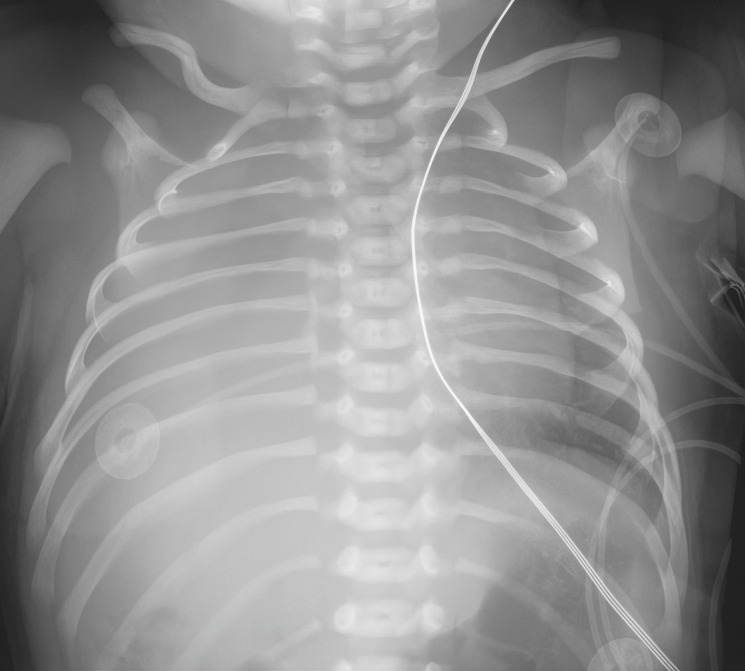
Single view chest X-ray performed on the first day of life. Complete opacification of the right chest is noted on chest film. The left chest shows a left-shifted heart border with decreased peripheral lung markings. Chest X-ray was read as “near-complete opacification of the right lung, favored atelectasis/collapse.”
